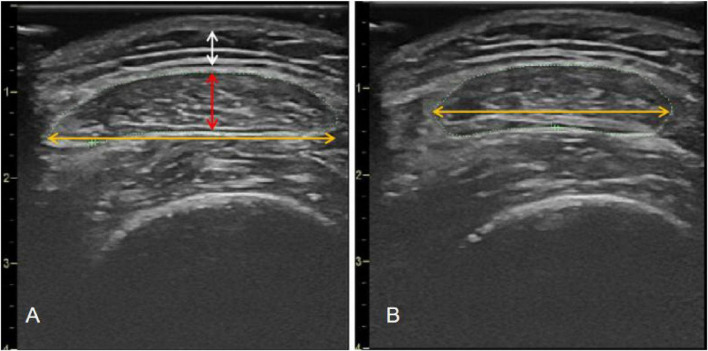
Ultrasonic measurement of rectus femoris transverse diameter for calculating ΔRF-d (rectus femoris rest-contraction transverse diameter difference, resting diameter minus contraction diameter). (A) Resting rectus femoris; yellow double-headed arrow = resting maximum transverse diameter; red double-headed arrow = resting muscle thickness; green outline = resting cross-sectional area. (B) Rectus femoris under neuromuscular electrical stimulation contraction; yellow double-headed arrow = contracted maximum transverse diameter (shorter than resting diameter). ΔRF-d equals the difference between resting transverse diameter and contracted transverse diameter; a larger ΔRF-d represents stronger muscle contractile function.
